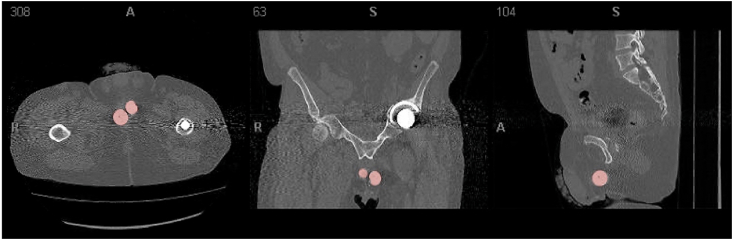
CT pelvis demonstrating suboptimal balloon position (red) position after the initial ProACT™ surgery. (For interpretation of the references to colour in this figure legend, the reader is referred to the Web version of this article.)
